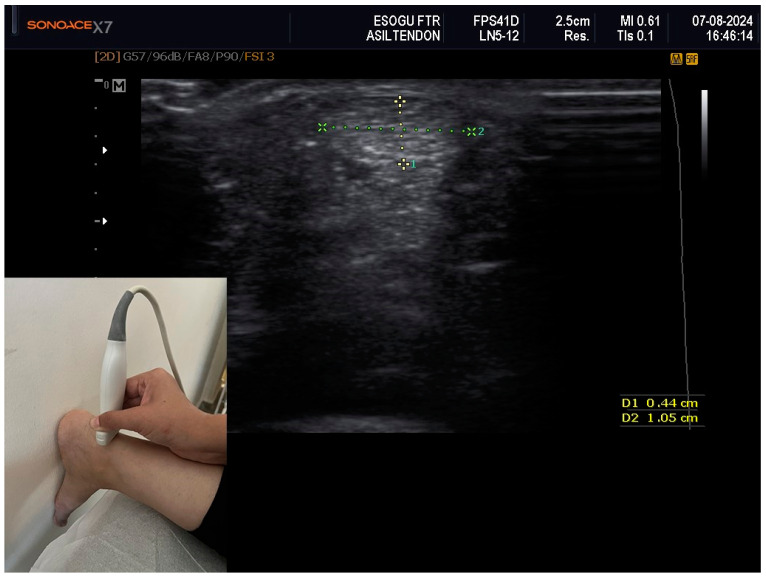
Ultrasound Imaging of the Achilles Tendon. The small image demonstrates the positioning of the ultrasound probe, placed perpendicular to the long axis of the Achilles tendon to obtain an axial plane at the level of the medial malleolus. The larger image depicts the measurements of the Achilles tendon, including thickness (anterior-posterior dimension, labeled as 1) and width (medial-lateral dimension, labeled as 2).
